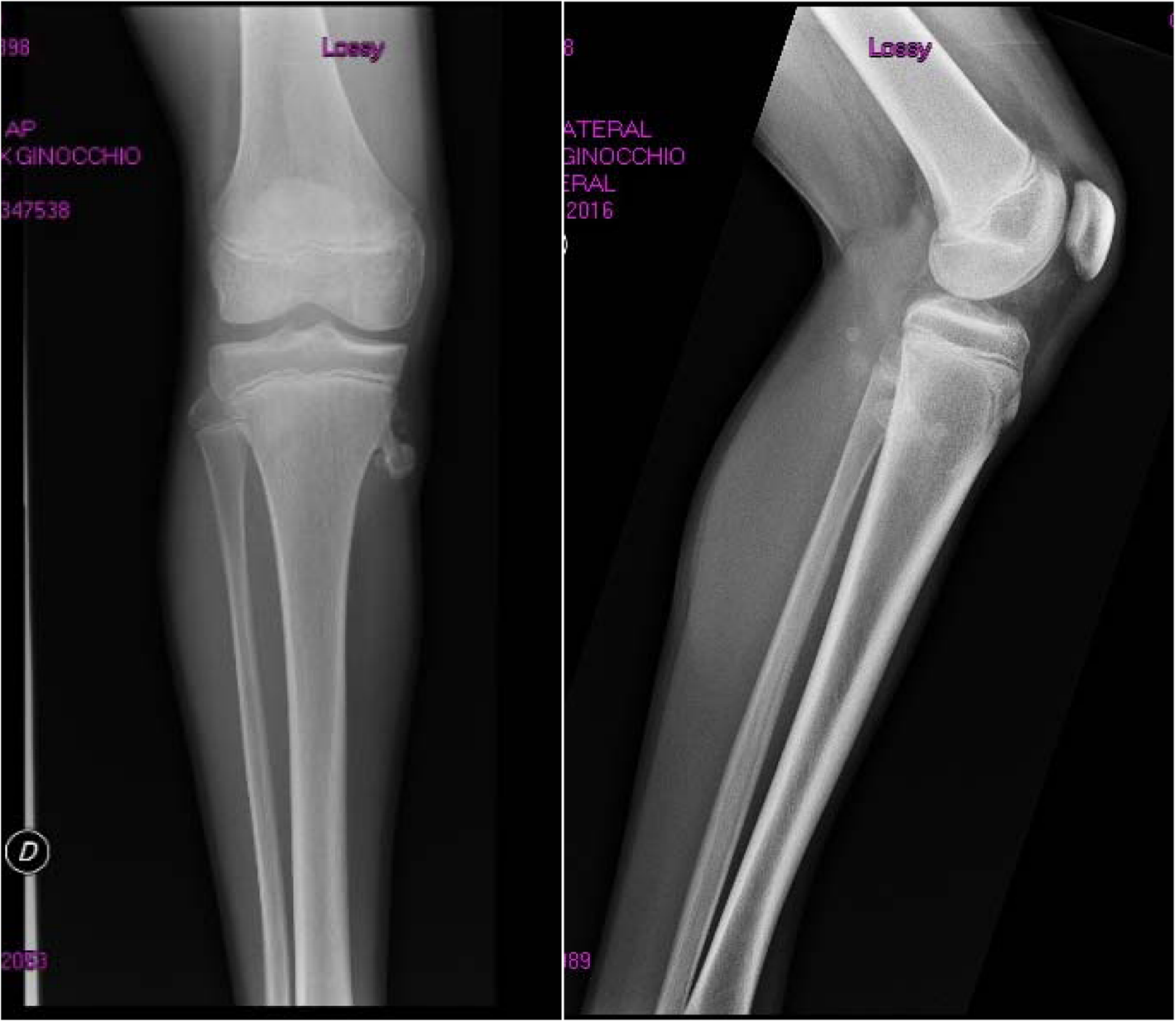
Patient 1 right leg X-rays during GH treatment
